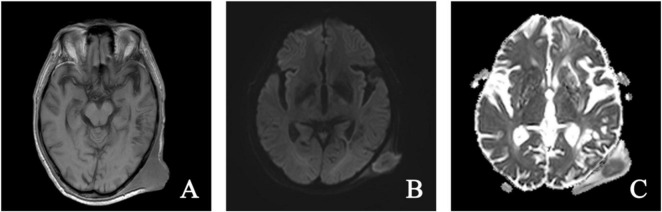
Magnetic resonance imaging axial T1-weighted (A), diffusion-weighted (B), and apparent diffusion coefficient (C) images. On T1-weighted imaging, a slightly hyperintense, round-shaped lesion was observed in the subcutaneous region of the left occipital area. The lesion exhibited high signal intensity on DWI and low signal intensity on the ADC. The internal signal of the lesion appeared heterogeneous, with an approximate size of 31 × 28 mm.
